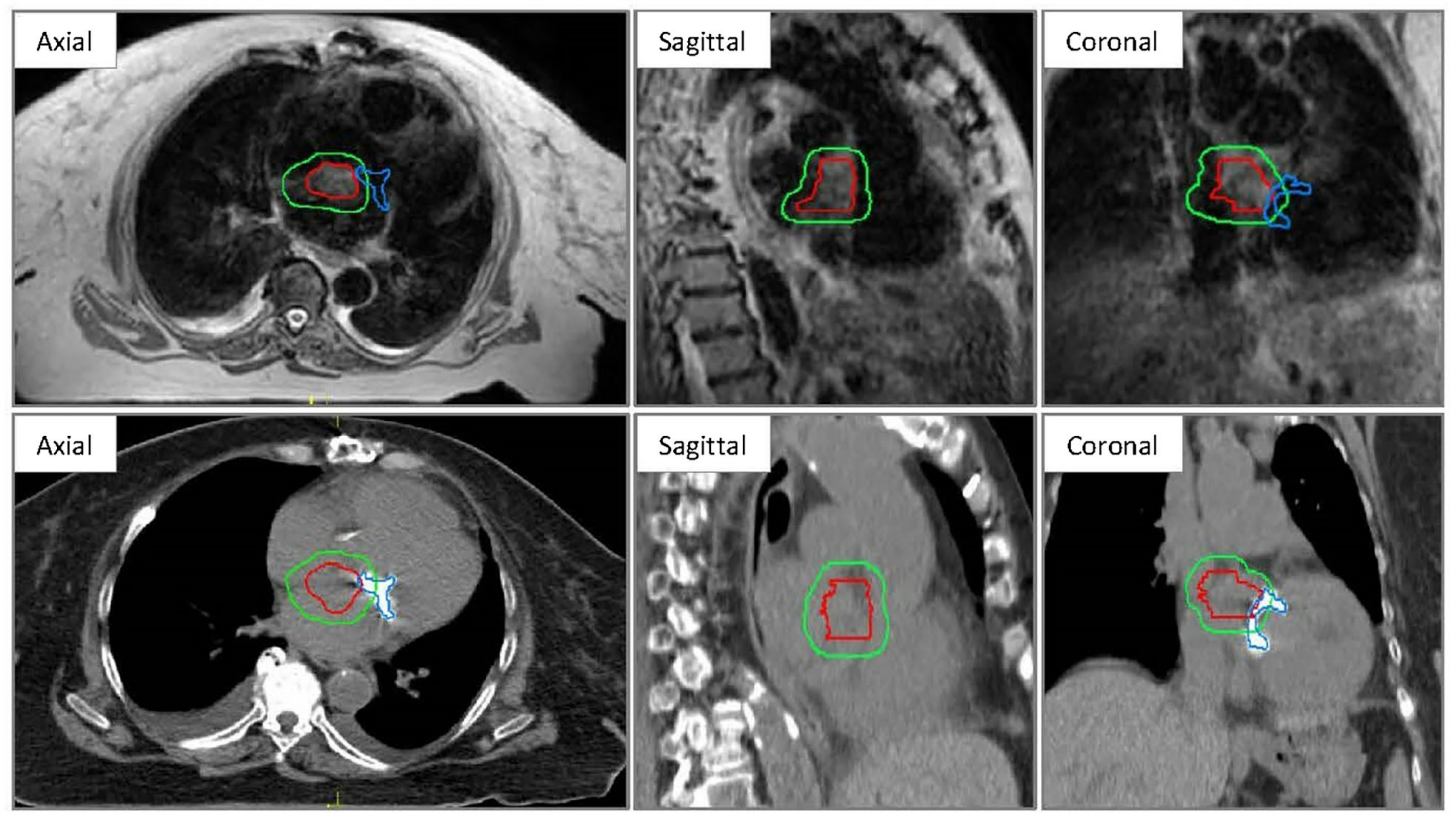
MR (top) and CT (bottom) reference scans showing gross tumour volume (red), planning target volume (green) and prosthetic valve (blue).
